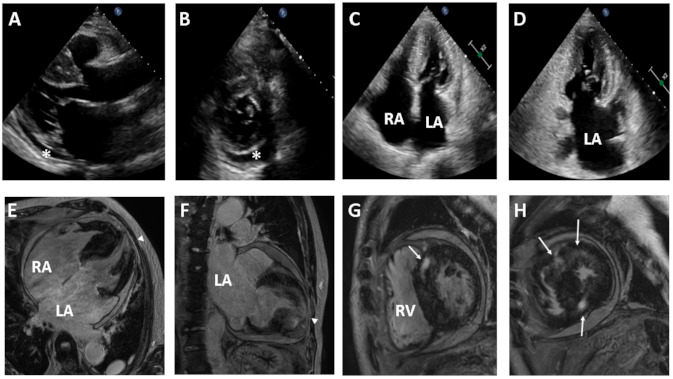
Transthoracic echocardiography (TTE) (upper panel) and contrast-enhanced cardiac magnetic resonance (CMR) images (lower panel) from the proband with idiopathic restrictive cardiomyopathy. Two-dimensional TTE reveals a normal left ventricle chamber (A), small pericardial effusion (asterisk) (B), and biatrial enlargement (C,D). CMR images demonstrate biatrial enlargement and transmural late gadolinium enhancement (LGE) in the apical wall (arrowhead) (E,F) and mid myocardial patchy LGE in the ventricular septum (arrow) (G,H). LA, left atrium; RA, right atrium; RV, right ventricle.
